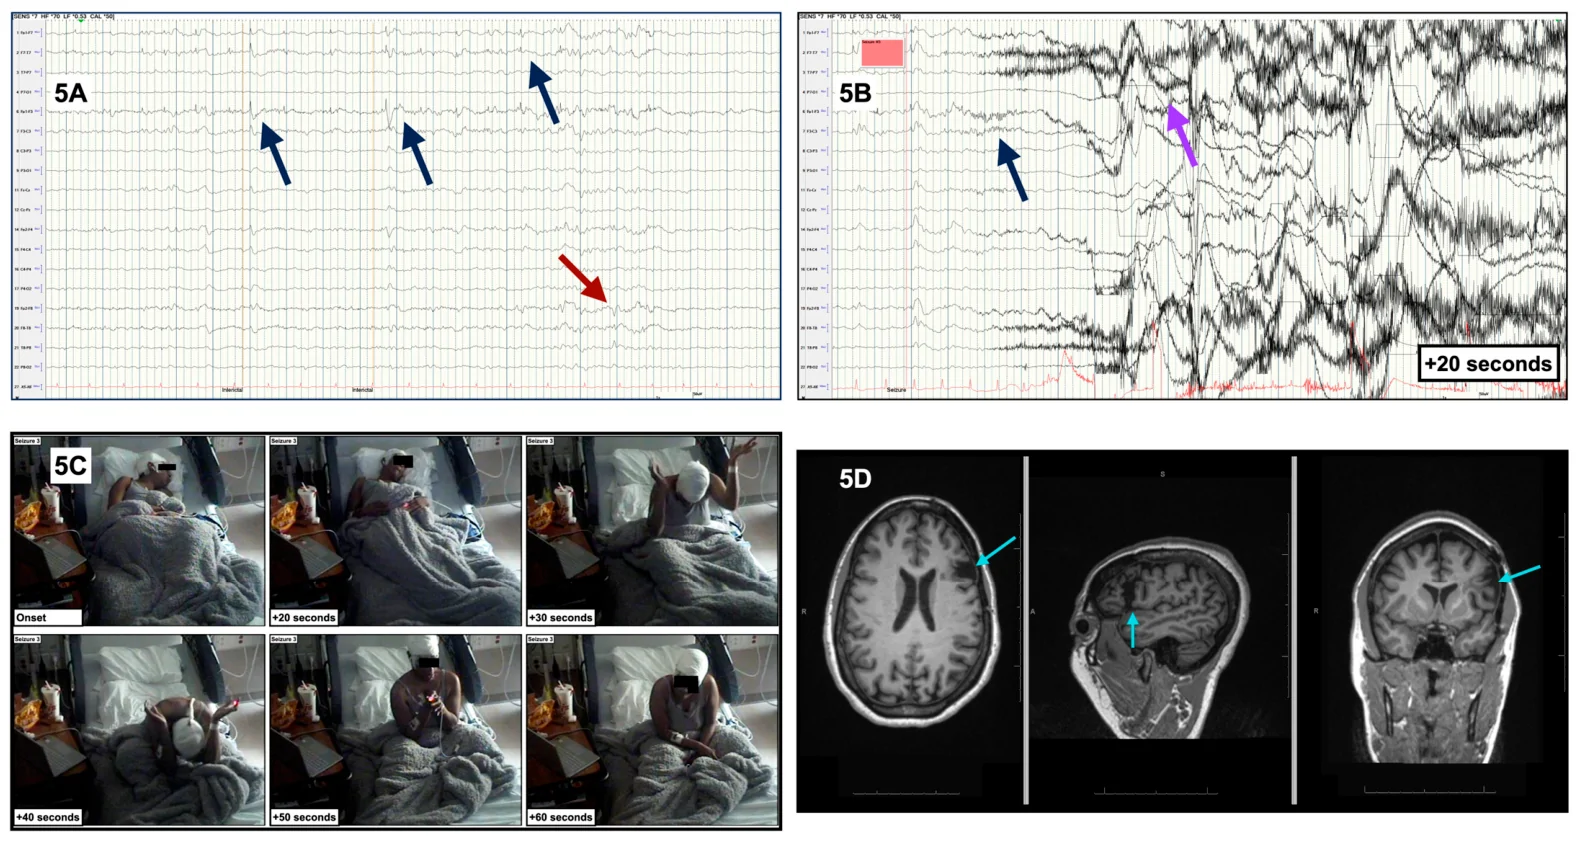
(A–D) Case 2—Scalp EEG findings, semiology, and postoperative brain MRI. (A) is an EEG reviewed in an AP bipolar montage (sensitivity 7 µV, high-frequency filter 70 Hz, low-frequency filter 0.53 Hz) revealing interictal epileptiform activity within the left frontal region (dark blue arrows) and right temporal region (red arrows). (B) is the third seizure recorded during video-EEG monitoring on scalp EEG in an AP bipolar montage (sensitivity 7 µV, high-frequency filter 70 Hz, low-frequency filter 0.53 Hz). There is lower-amplitude, rhythmic beta-range activity (dark blue arrow) within the left fronto-central region. After this, the EEG is largely obscured by a myogenic artifact (purple arrow). In (C), screenshots of the ictal semiology are depicted. At +20 s, there is an arousal from sleep. By +30 s there are gestural movements of the upper extremities followed by flexion at the waist (+40 s), clasping of both hands at +50 s, and behavioral arrest at +60 s. These features are suggestive of frontal lobe seizures and specifically seizures arising from the prefrontal cortex [33,43,45,46,47]. (D) is a postoperative brain MRI obtained after the initial resective surgery completed at an outside institution. Note: An MEG was not obtained in the initial epilepsy presurgical evaluation.
